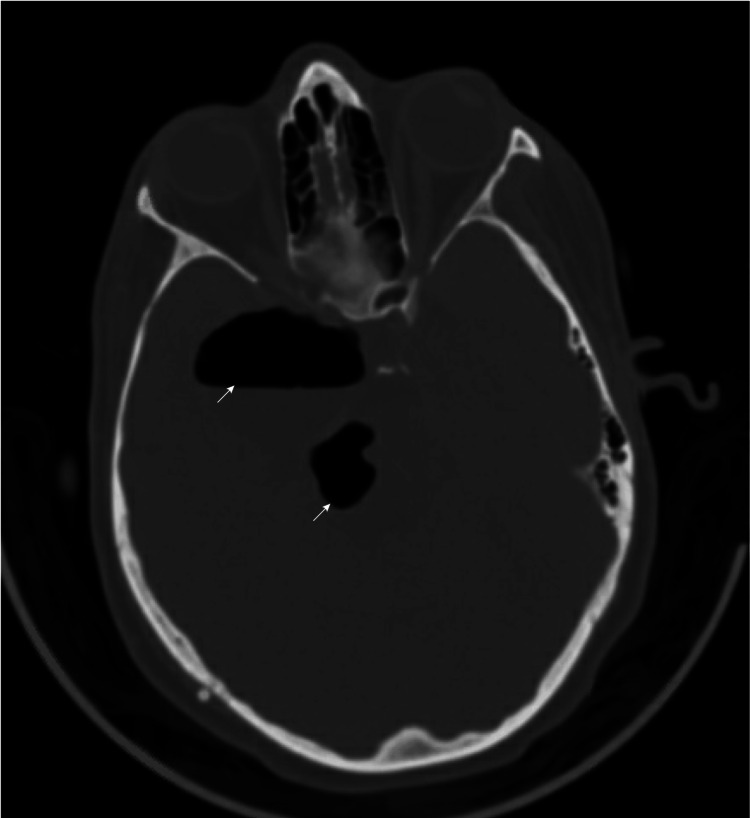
CT imaging demonstrating localized pneumocephalus within the tumor cavity
